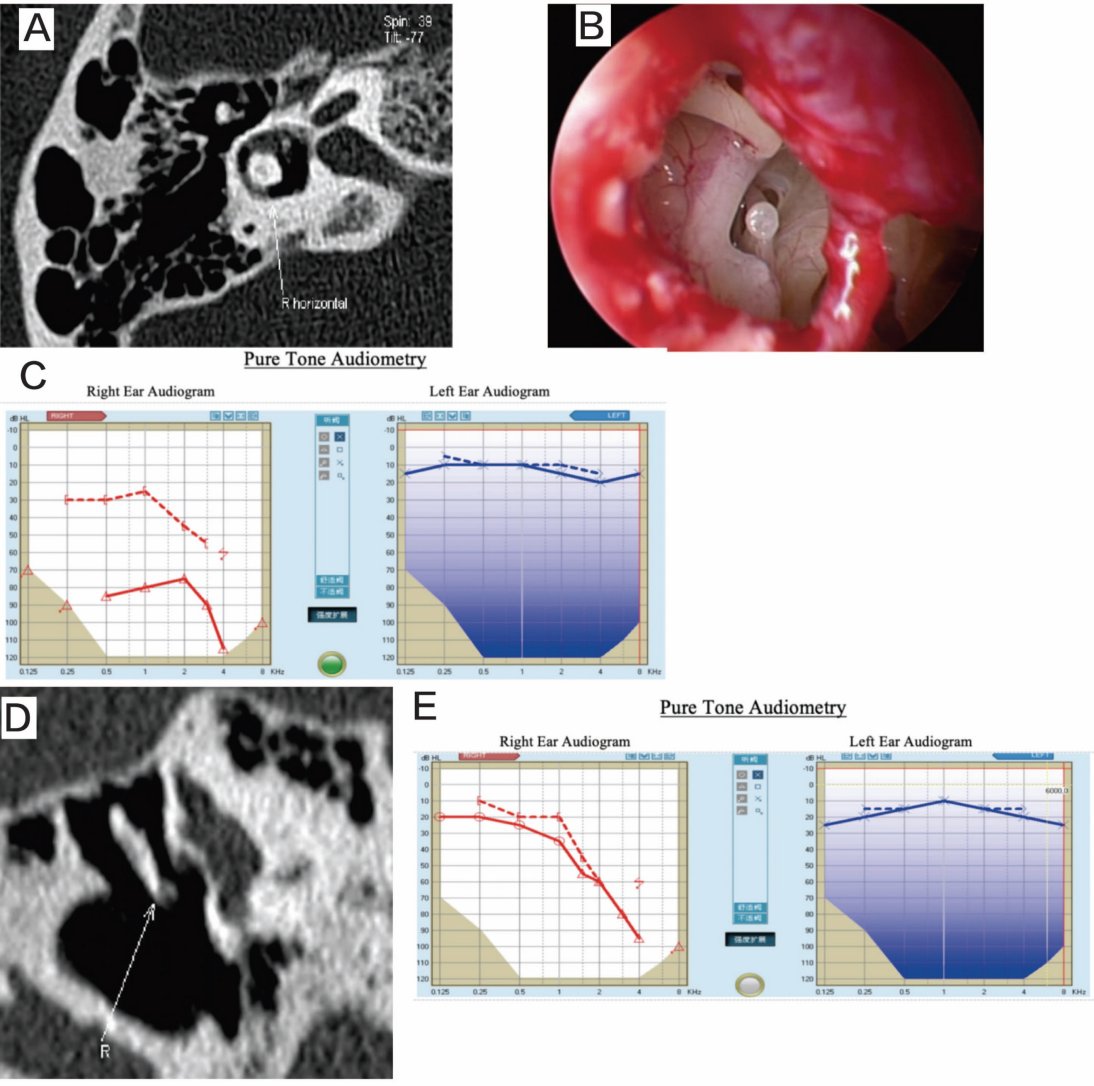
Patient 12. A: preoperative CT, revealing pneumolabyrinth. B: the deep depression of the stapes into the vestibule. C: preoperative audiogram. D: postoperative HRCT, showing that the pneumolabyrinth has been resolved. E: postoperative audiogram.
